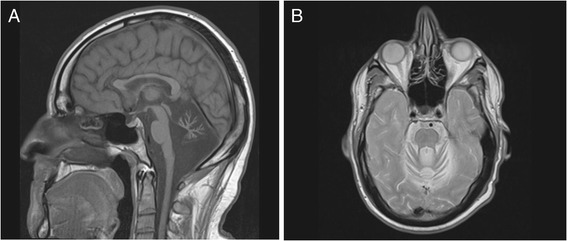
Cerebral MRI (1.5 Tesla). (A) Cerebral MRI (T1 serie, midline sagittal) of the proband in Family 1 at the time of investigation. Severe atrophy of the whole cerebellum and the anterior part of the corpus callosum. (B) Same examination, but T2 axial scan at the level of the superior cerebellar peduncle. There is an atrophy of both cerebellar hemispheres with widened sulci, and vermis atrophy. The fourth ventricle is moderately dilated. There are a few diffuse hyperintensity signals in the brainstem. The cerebral hemispheres look normal.
